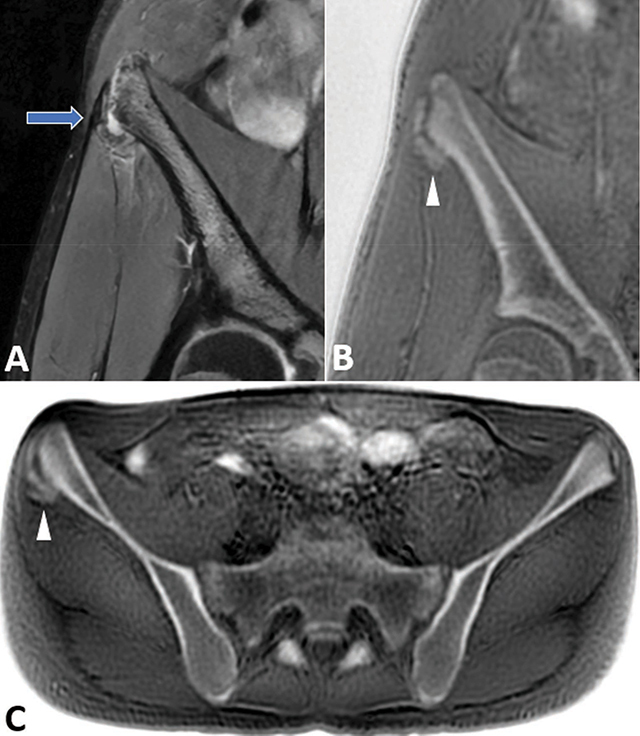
Coronal PDFS MR image shows lateral avulsion of the ossification center (A, arrow). Coronal and axial oZTEo images show the osseous displaced fragment with periosteal reaction (B-C, arrowhead).
